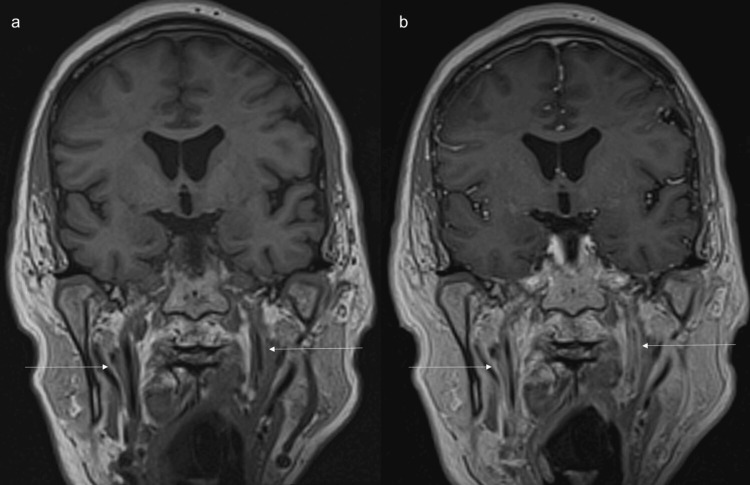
MRI shows long-segment abnormal wall thickening and enhancement of cervical portion of internal carotid arteries. (a) Coronal non-contrast and (b) contrast enhanced T1 space sequences show long segment abnormal wall thickening and enhancement of cervical portion of internal carotid arteries(arrows).
